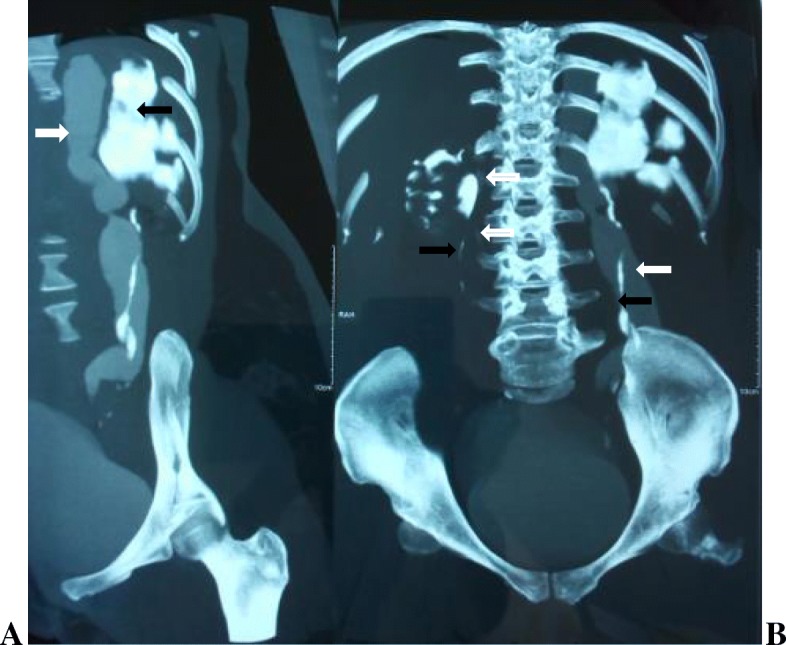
Excretory phase of computed tomography scan. a A 3/4 left view showing the upper pole moiety (*white arrow*) and the lower pole moiety (*black arrow*). b Bilateral duplex collecting system with hydroureteronephrosis of the left upper collecting system. The upper pole moieties and their ureters are poorly opacified (*white arrows*) especially on the right. The *black arrows* show the lower pole moiety ureters
